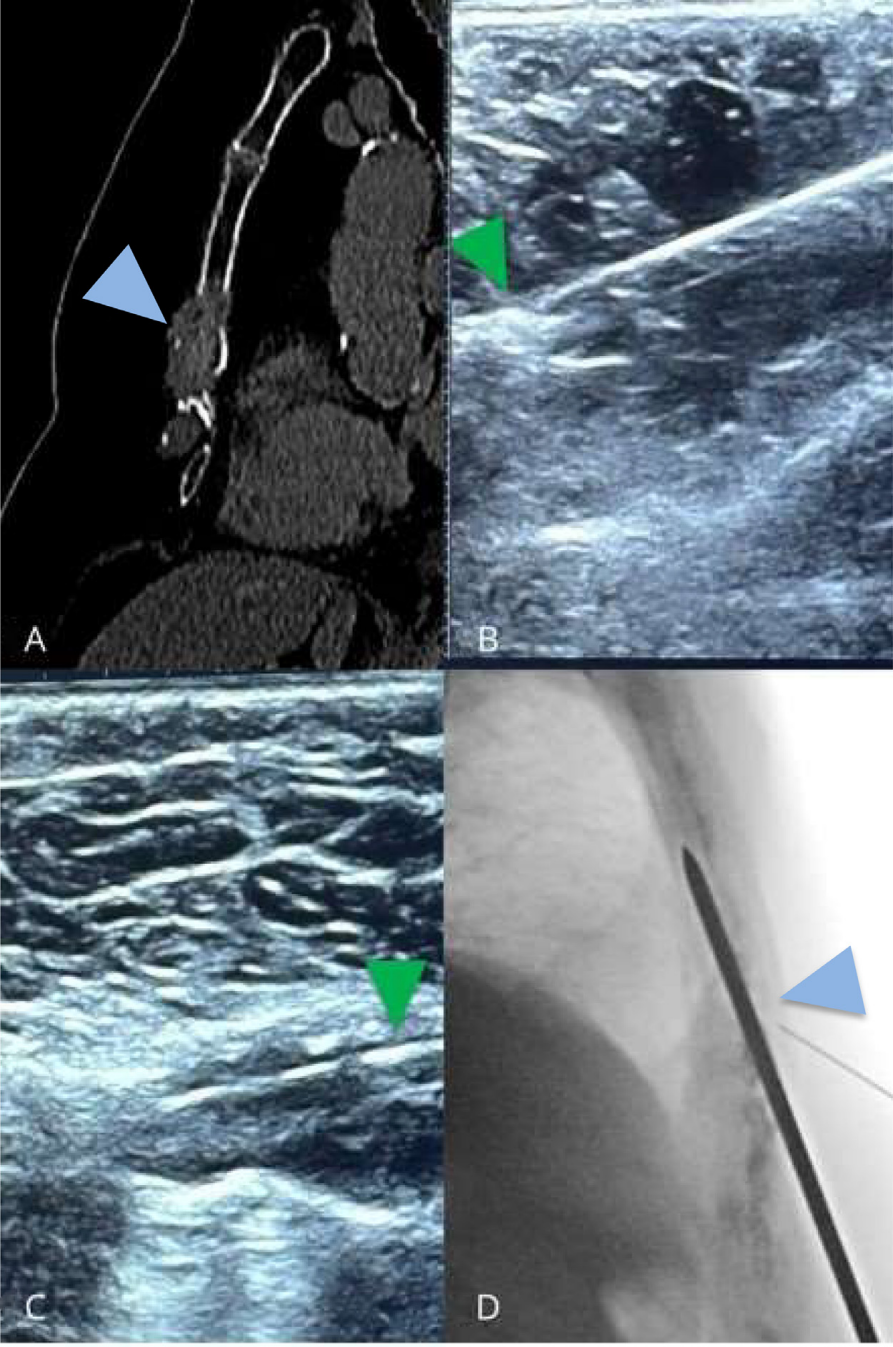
Diagnostic preoperative CT scan showing a large lytic lesion in the inferior half of the sternum with microfractures across the lesion (Blue Arrowhead). (A) 8G bone trocar visible under ultrasound guidance in contact with healthy bone (Green Arrowhead). (B) Visibility of the trocar into the lytic lesion under ultrasound (Green Arrowhead). (C) Lateral fluoroscopy confirming correct positioning of the trocar and 22G marker needle (Blue Arrowhead) (D).
